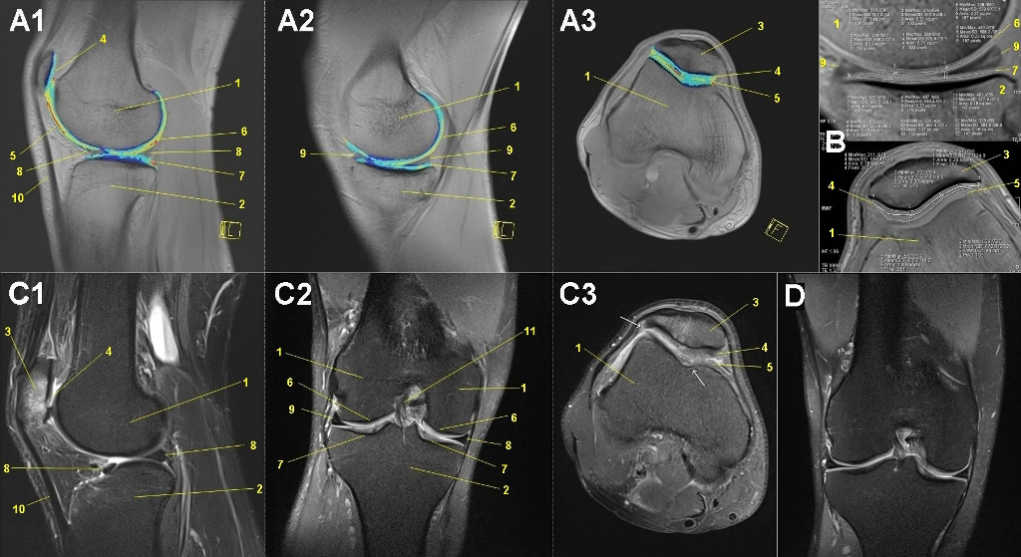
Mobile MRI of the knees. Femoral condyle (1), tibial head (2), patella (3), retropetallar cartilage layer (4), ventral femoral cartilage layer (5), dorsal femoral cartilage layer (6), tibial cartilage layer (7), medial meniscus (8), lateral meniscus (9), patellar tendon (10). A: female, 45-years-old, stage 44, 3,021 km (fused colored T2* GRE map: syngo™ MapIt fusion technique, A1: medial femorotibial joint sagittal, A2: lateral femorotibila joint sagittal, A3: femoropatellar joint transversal). B: male, 25-years-old, before start of TEFR09 Cartilage layer segmentation (T2* GRE map: syngo™ MapIt). C: male, 43-years-old, stage 40, 2,738 km (PDw TSE fs, C1: sagittal, C2: coronal, C3: transversal): Severe arthrosis of the patellofemoral joint with retropatellar cartilage defects (4, 5) and wide subchondral bone edema of the patella (3), intrachondral signal alterations of the femoral (6) and tibial (7) cartilage layers. D: male, 26-years-old, stage 40, 2,738 km (PDw TSE fs coronal): Nondescript cartilage layers of femorotibial joint.
